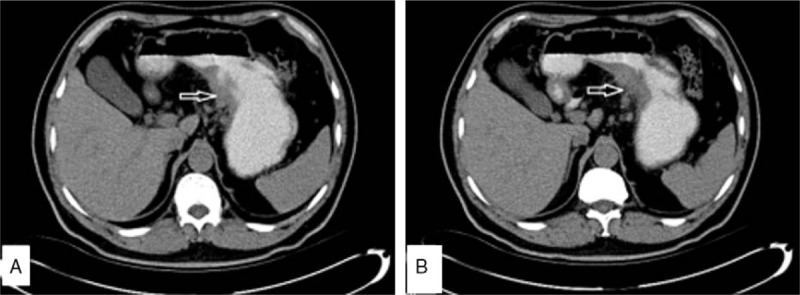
(A,B) CT scan showed wall thickness in the lesser curvature region of the stomach, and limited lymph nodes in the enterocoelia.
